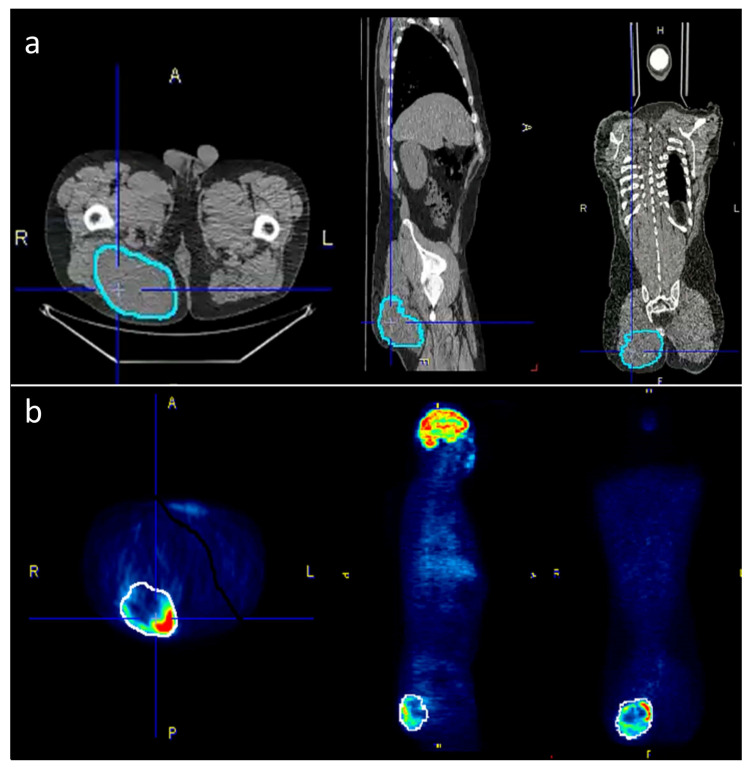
To accurately outline the tumors, the VOIs were selected semi-automatically on the PET-images where possible and then manually adjusted to the tumor borders on the CT scan. (a) The corrected VOIs were then copied back to the PET-scan (b) in order to obtain identical VOIs for both PET and CT data.
